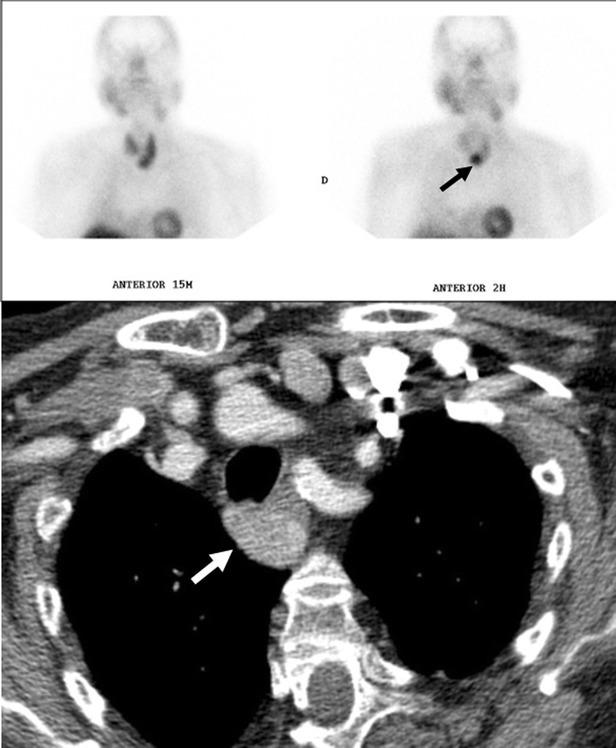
Parathyroid adenoma in a 66-year-old man with hypercalcaemia, hypophosphataemia and elevated PTH values. Tc-99m MIBI scan shows a focus of hyperactivity (*black arrow*) adjacent to the lower pole of left thryoid lobule. Enhanced-CT scan shows a superior mediastinal enhanced mass (*white arrow*)
